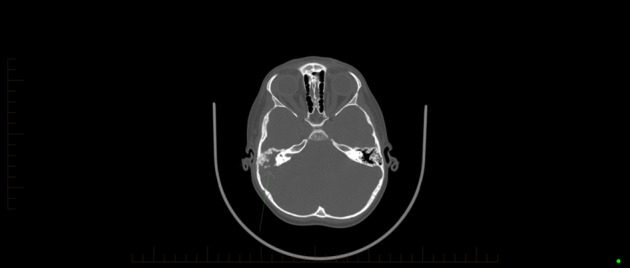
Initial CT imaging demonstrating ossified right middle ear and mastoid air cells, bony irregularity and cortical destruction consistent with osteomyelitis.
